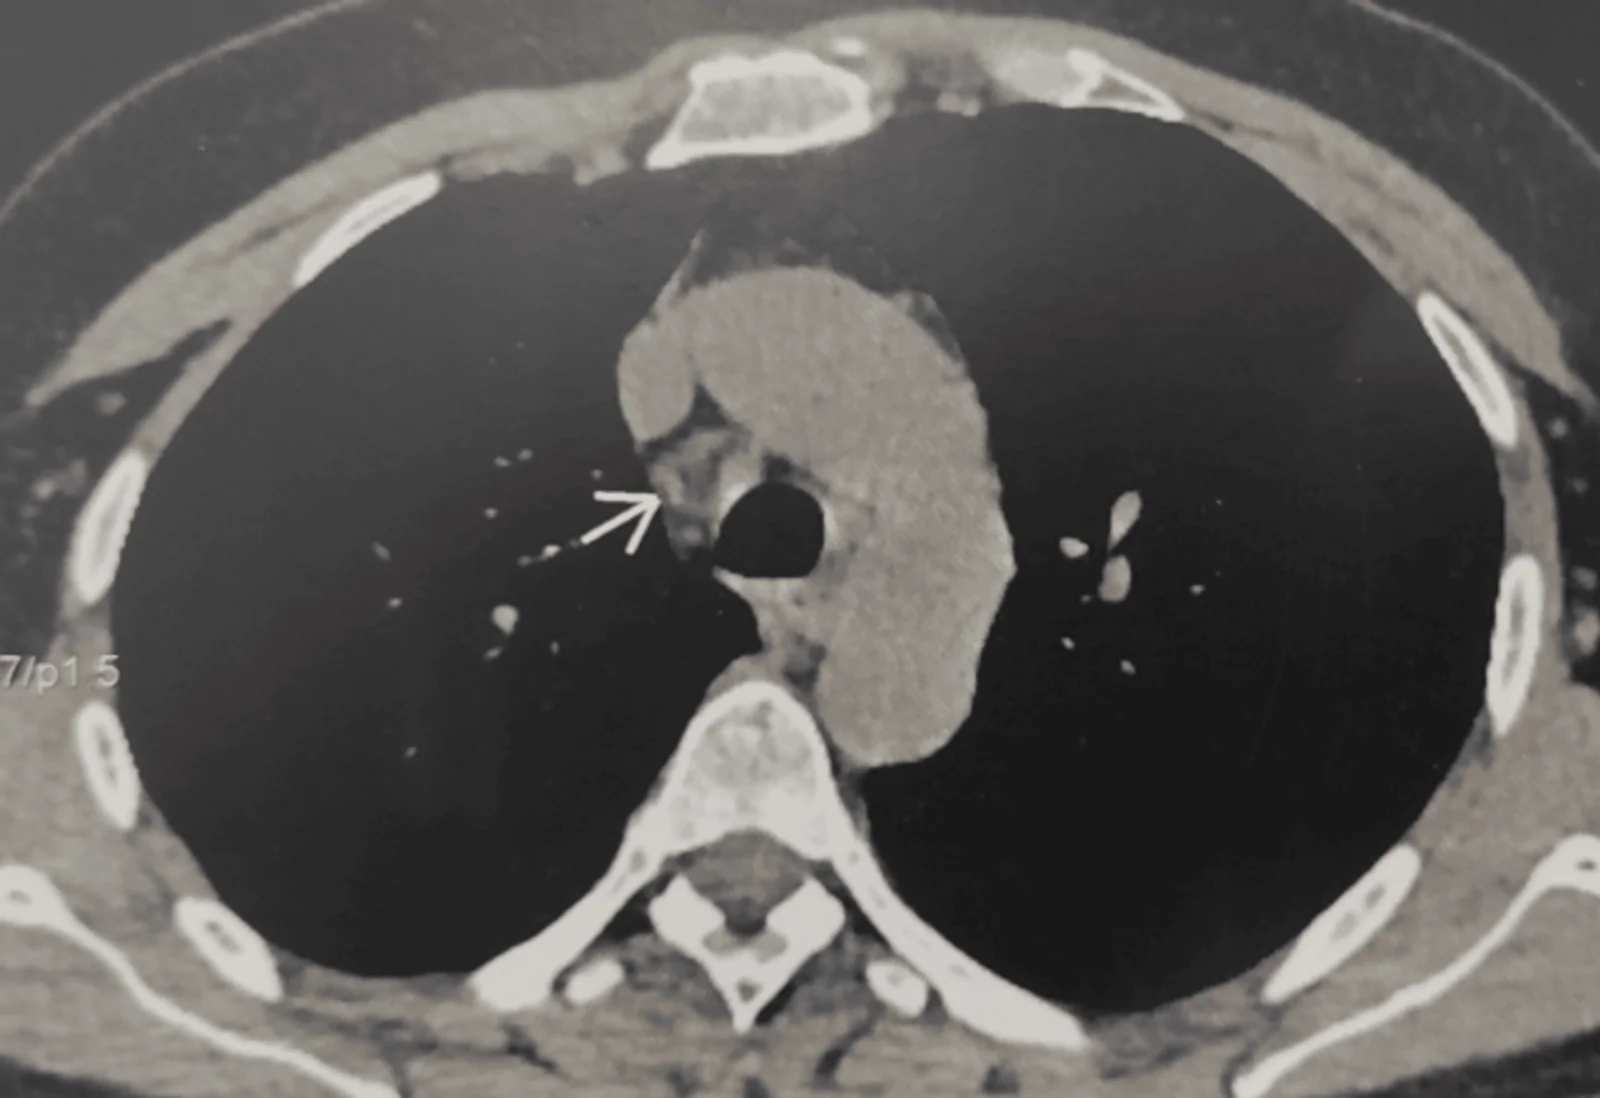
Follow-up chest computed tomography (CT) scan (2025, mediastinal window) Persistent mediastinal lymphadenopathy (white arrow)
